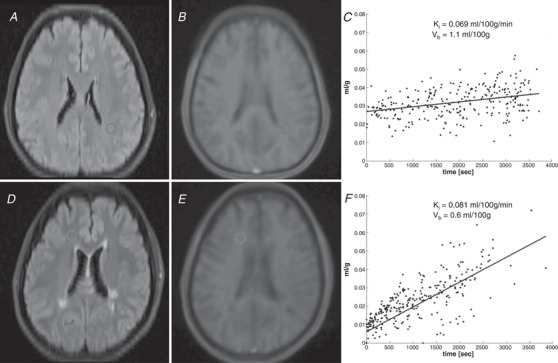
Region of interest (ROI) placement and Patlak plots *A*, axial fluid‐attenuated inversion recovery sequence (FLAIR) in a control subject with manual ROI placement in the normal‐appearing white matter. *B*, first dynamic frame from the same subject, with ROI transposed. *C*, Patlak plot derived from the ROI. *D–F*, same images for a subject with relapsing–remitting multiple sclerosis. ‘Time’ in the *x*‐axis of the Patlak plots is normalized to arterial concentration.
